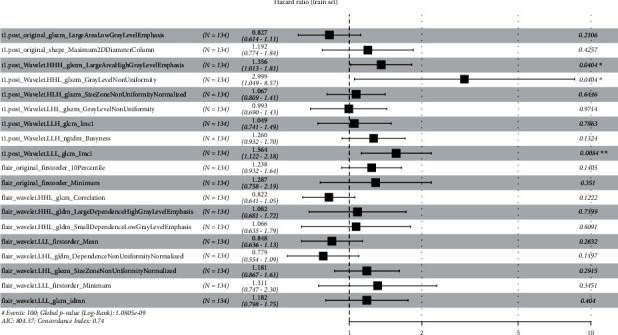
HR values with 95% CI and *p* values of each selected feature of the radiomics model on the forest map in the training set. Postcontrast axial T1-weighted (CE-T1) = t1.post; T2-weighted fluid attenuation inversion recovery (T2FLAIR) = flair.
